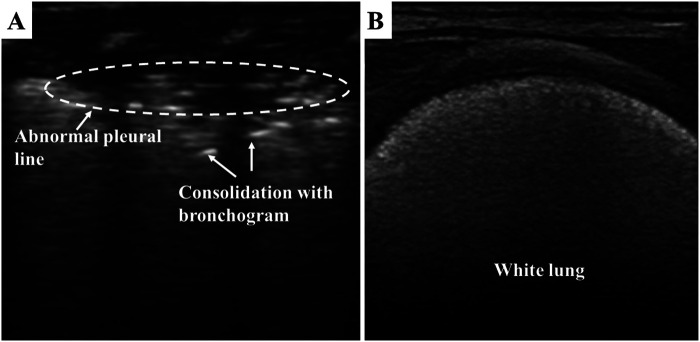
Respiratory distress syndrome on lung ultrasonography. (A) Pleural line is thick, irregular, or blurred. Lung consolidation with the air bronchogram is the most typical appearance of neonatal RDS. (B) white lung is derived from the emerged compact B-lines.
